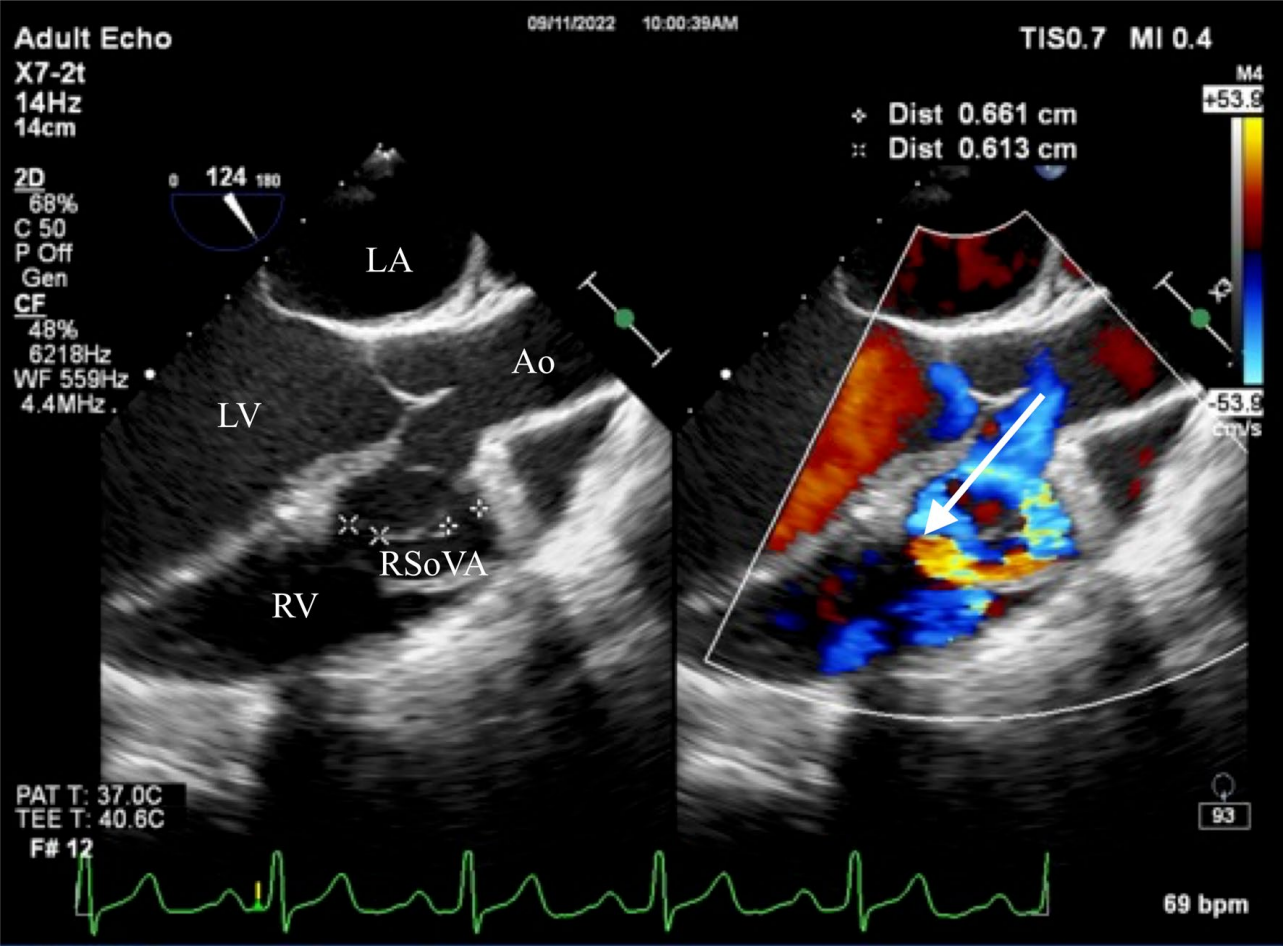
Transoesophageal echocardiography showed rupture of the sinus of Valsalva aneurysm in the RCC region (diameter 1.5 cm) with multi fenestrated (diameter 0.61 to 0.66 cm) towards RV (arrow). Ao = aorta; RV = right ventricle; LV = left ventricle; LA = left atrium; RSoVA = ruptured sinus of Valsalva aneurysm
